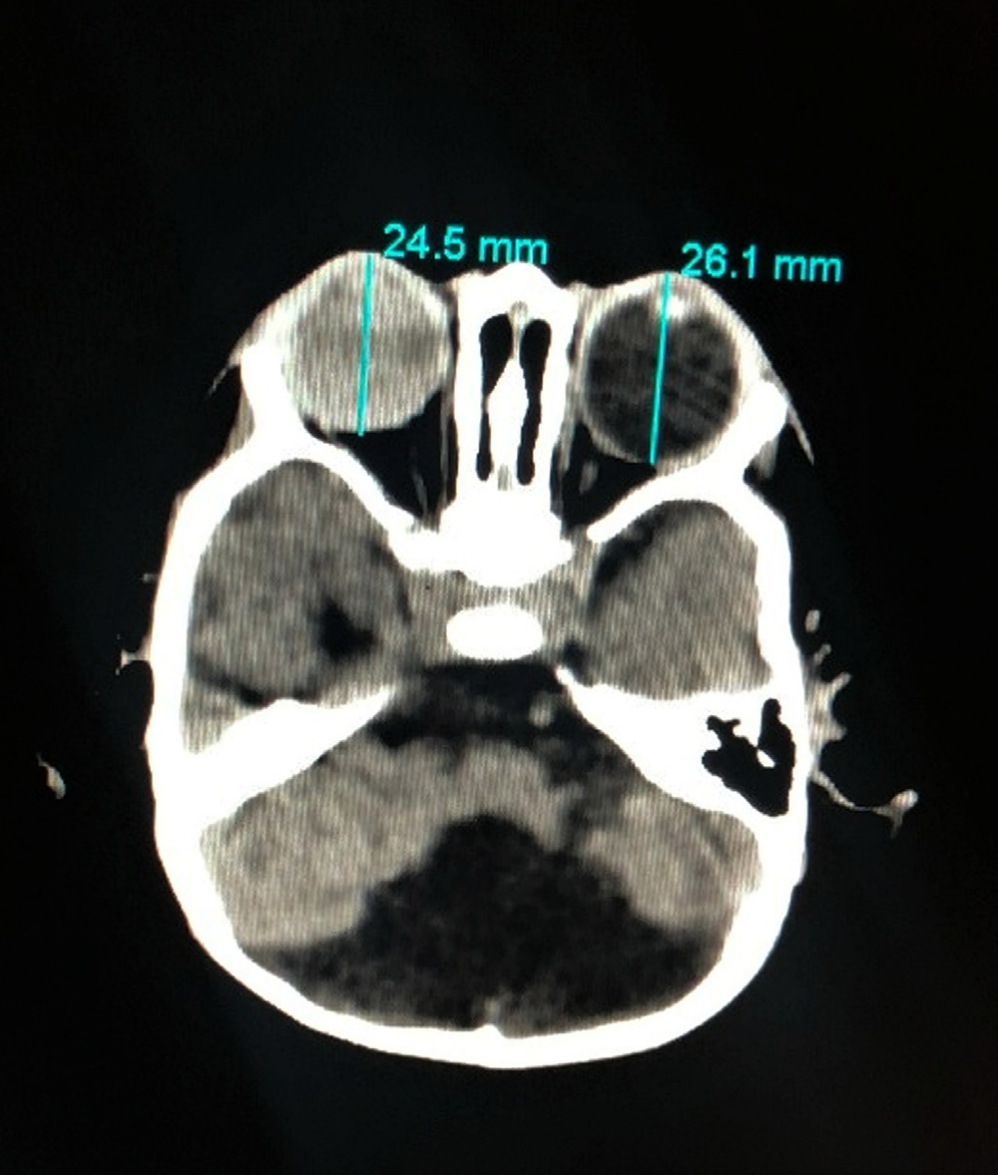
(yellow arrow): Axial CT image shows midline open communication of the fourth ventricle with a large cystic posterior fossa. The cerebellar hemisphere is hypoplastic representing dandy walker malformation (purple arrow): The temporal horns of the lateral ventricle are also dilated
